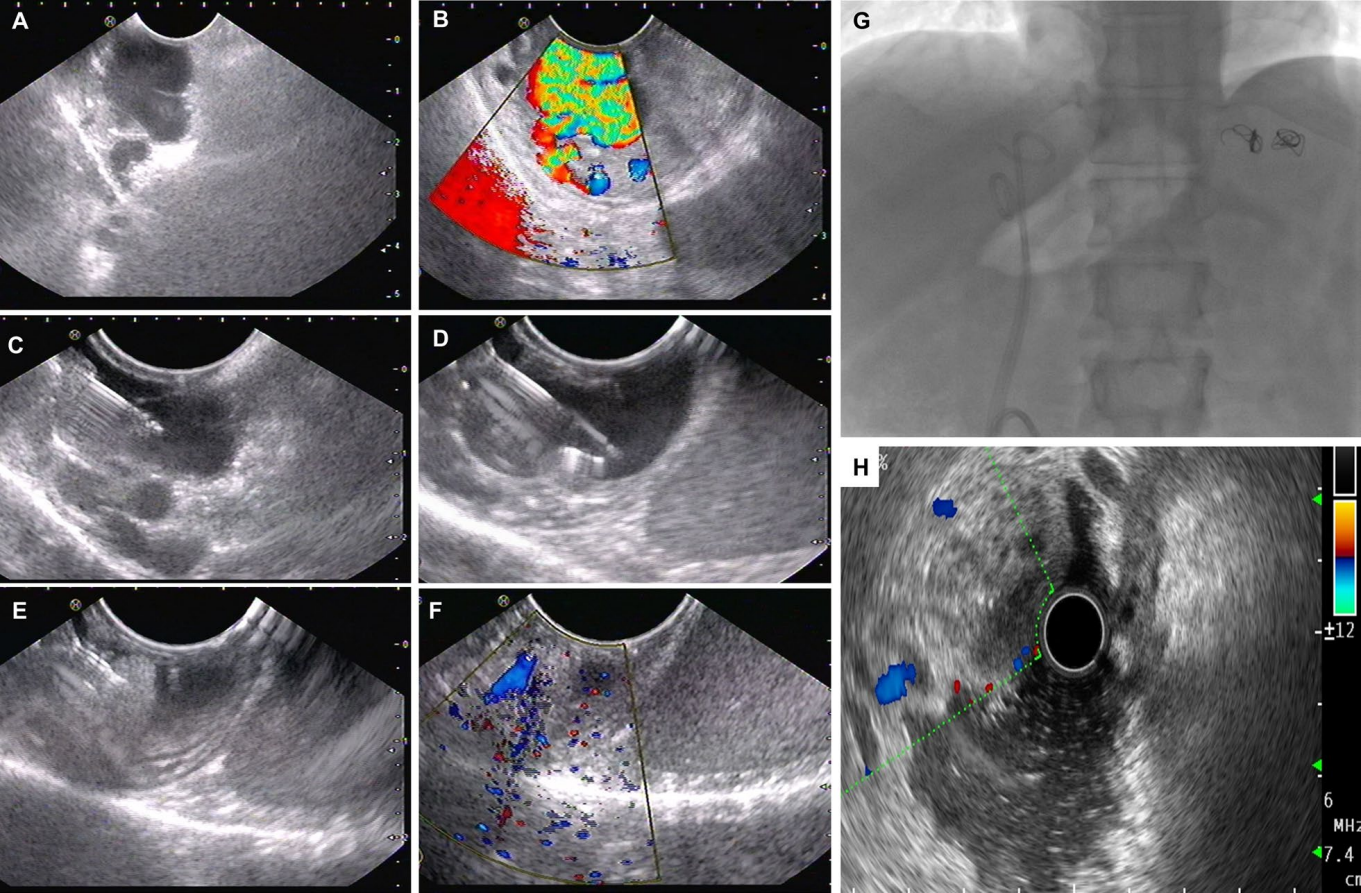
Endoscopic ultrasound (EUS) guided coiling and glue therapy for recurrent bleeding from gastric varices. Large gastric varix is noted (a) with turbulent blood flow within (b). A 22 G aspiration needle is pushed into the varix (c) and coils inserted into the varix lumen under direct vision (d). Additionally, 1.5 mL of cyanoacrylate glue is injected to completely obliterate the variceal lumen (e). Immediate post treatment, the turbulent flow has almost disappeared from variceal complex (f). Six months after treatment, the coils are visible on abdominal fluoroscopy (g) and there is complete obliteration with loss of Doppler signals from the varix on repeat EUS (h)
